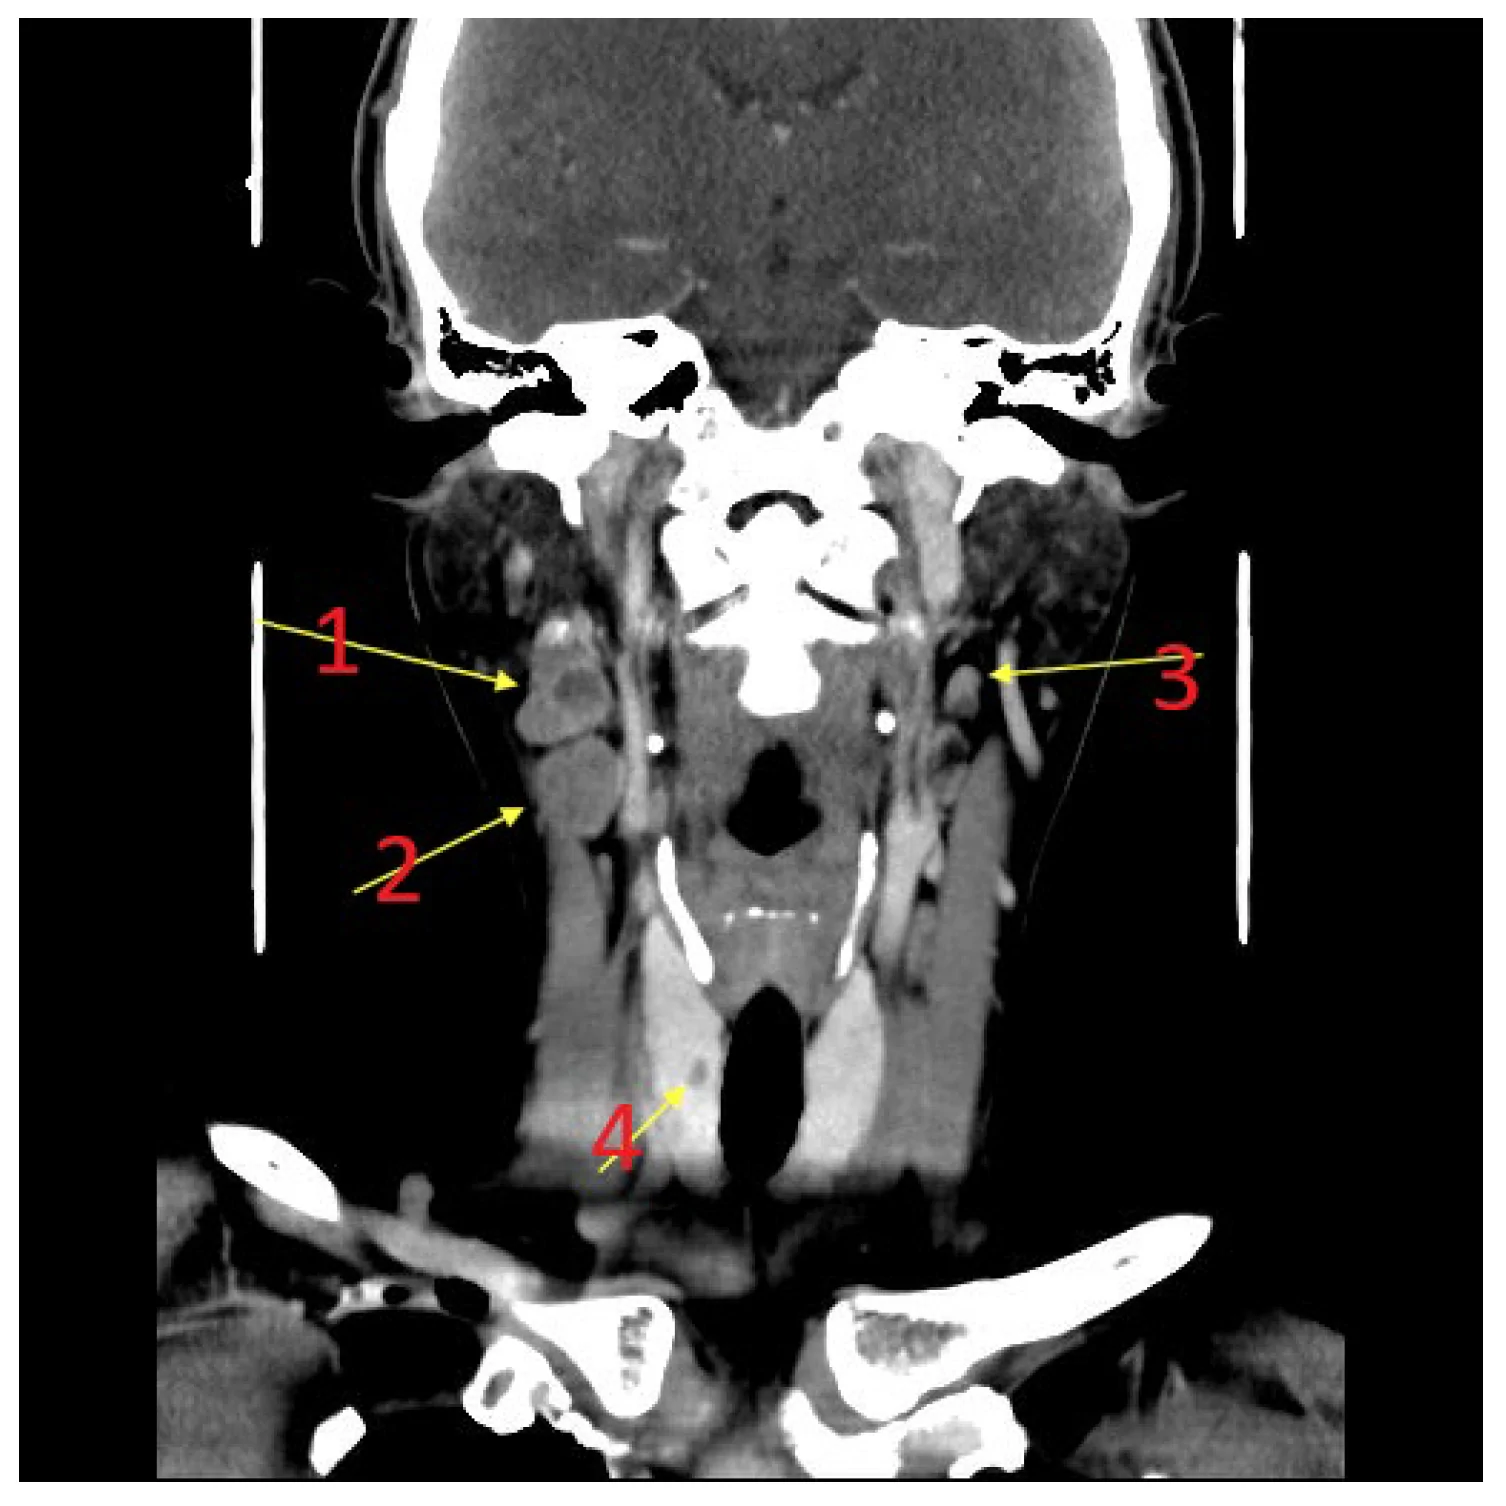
Contrast-enhanced coronal CT of the neck demonstrating enlarged cervical lymph nodes in the right level II (arrow 1), right level III (arrow 2), and left level II (arrow 3) regions. Arrow 4 indicates a small incidental thyroid nodule.
